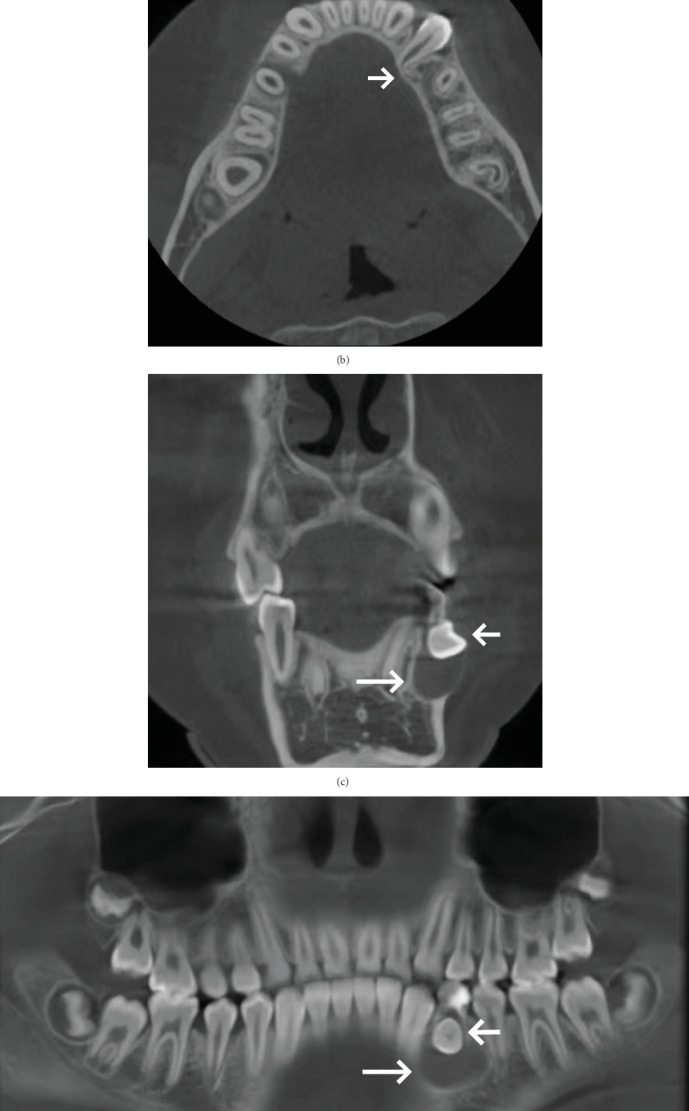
(a–e) Pretreatment CBCT examination: CBCT showed Tooth 74 apical areas with an oval transmission of about 11 × 7 mm (long arrow), the germ of permanent Tooth 34 was inclined in the buccal direction, impacted horizontally, and the root was curved (short arrow).
